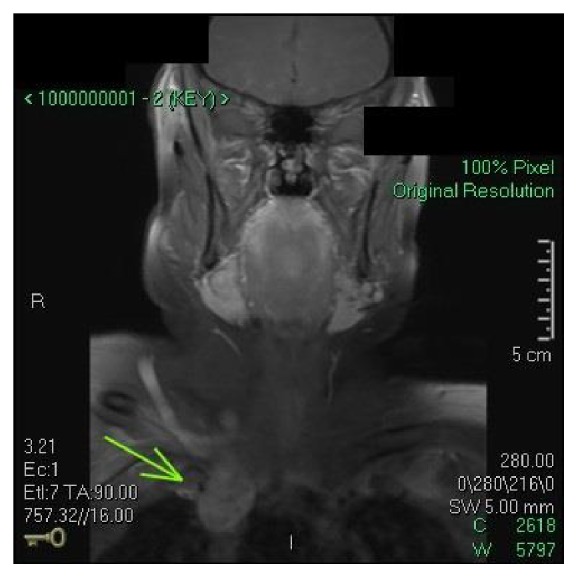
Coronal section from the preoperative magnetic resonance images showing a superior vena cava mass, presumed to be lymph nodes abutting the vein.
